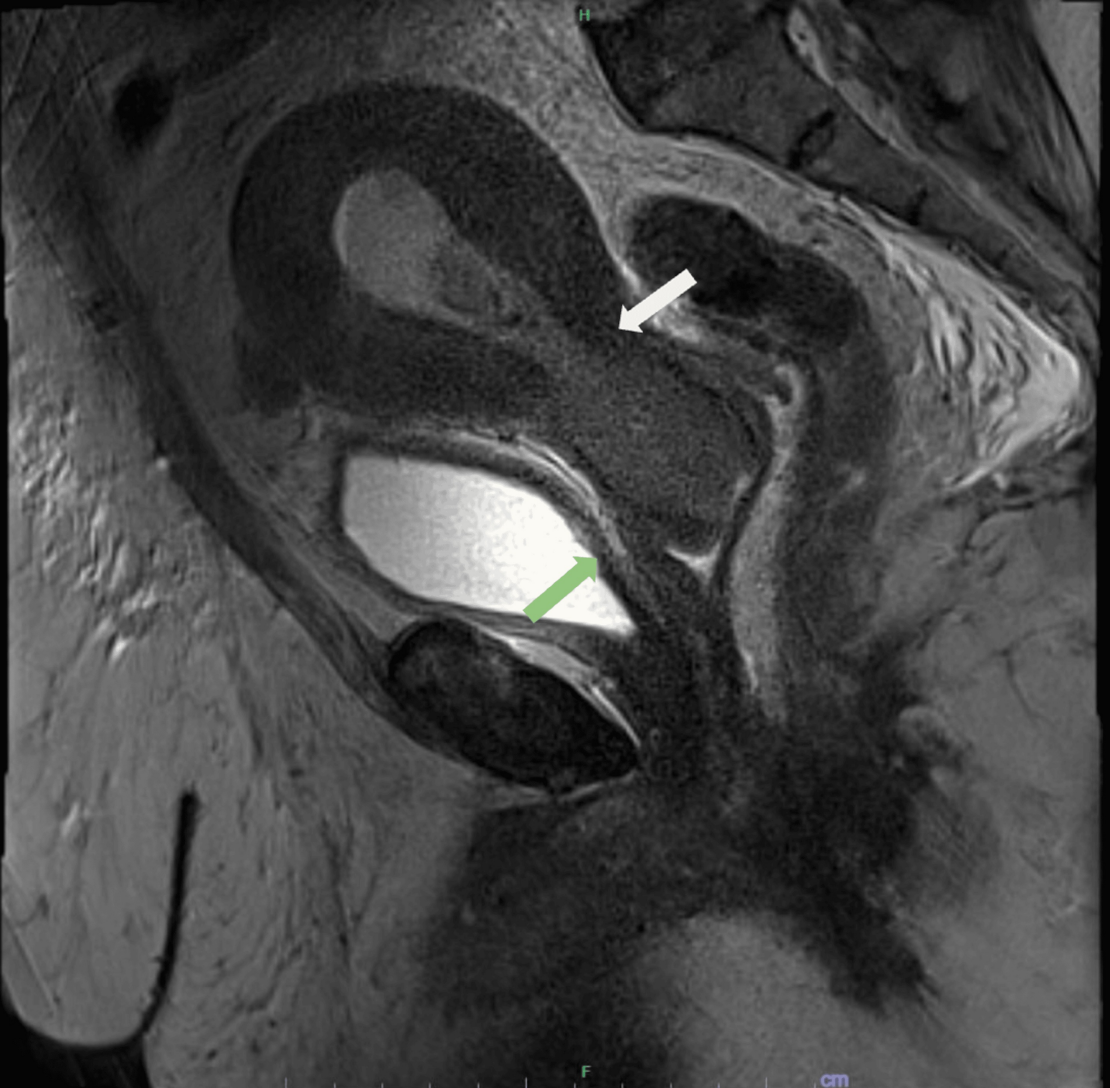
Sagittal view of T2-contrasted MRI of the pelvis The image shows cervical endometrial malignancy with cervical stroma invasion (white arrow) along with thickened peritoneum (green arrow) MRI: magnetic resonance imaging
